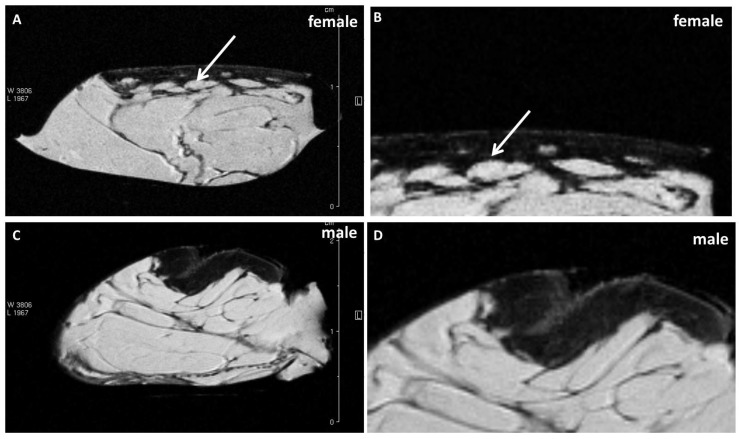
Cellulite MRI. (A,B): Cellulite of female patients. (C,D): Cellulite of male patients. The figure shows the organization of collagen fibers in the dermis of female and male. It is possible to observe a non-uniform distribution of collagen bundles both in female and in male. The main difference is represented by the dimension of adipose lobules surrounded by collagen fibers, larger in men and better defined by collagen septa. Moreover, the presence of dermal papillae was detectable only in the female dermis and not in male, indicating a different organization that could be related with cellulite development (A,B).
